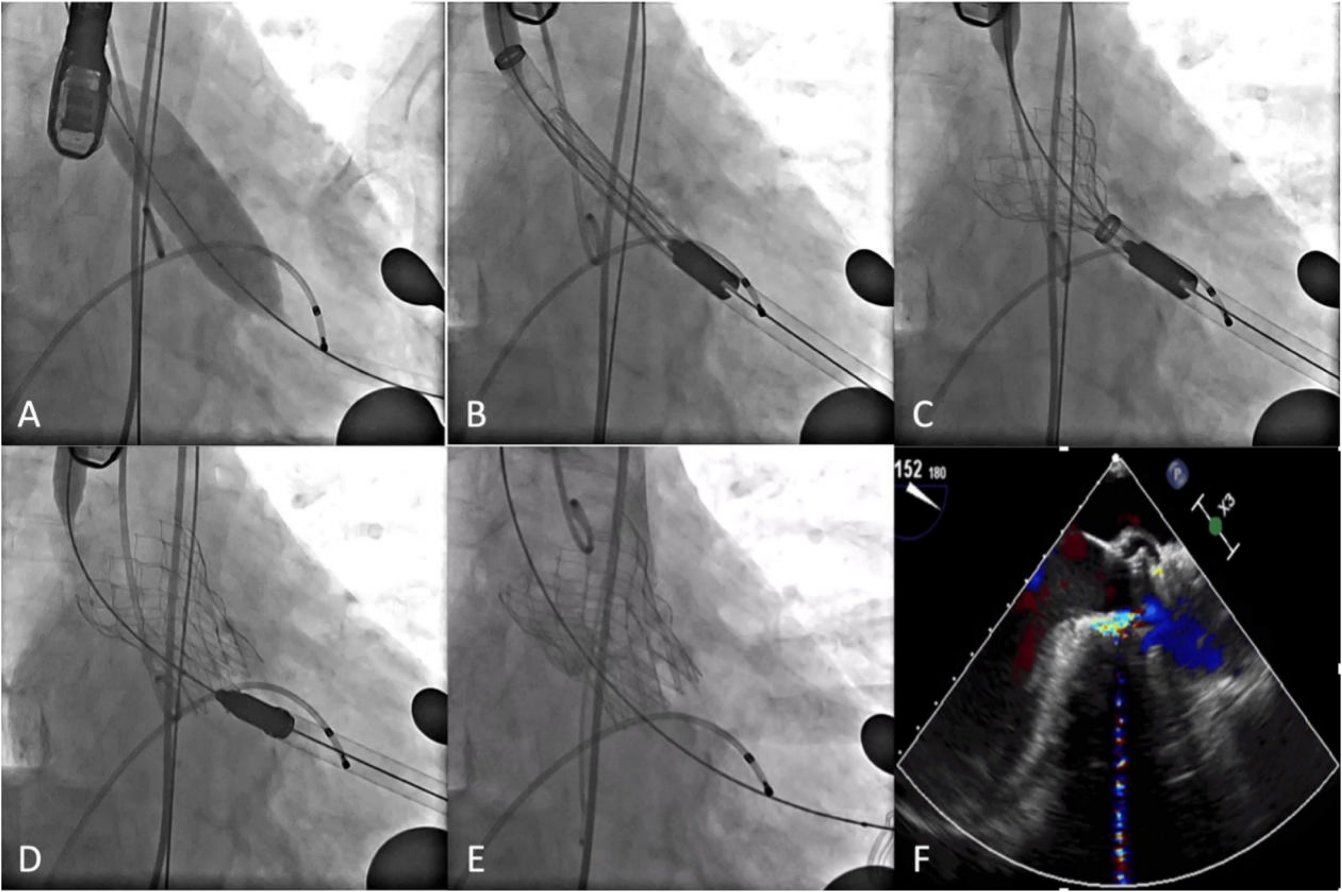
Procedural details. (A) Pre-dilation. (B) Xcor system was positioned. (C) The supporting arms were released. (D) The prosthesis was fully unfolded. (E,F) DSA and transesophageal echocardiography showed the position and morphology of the prosthesis.
